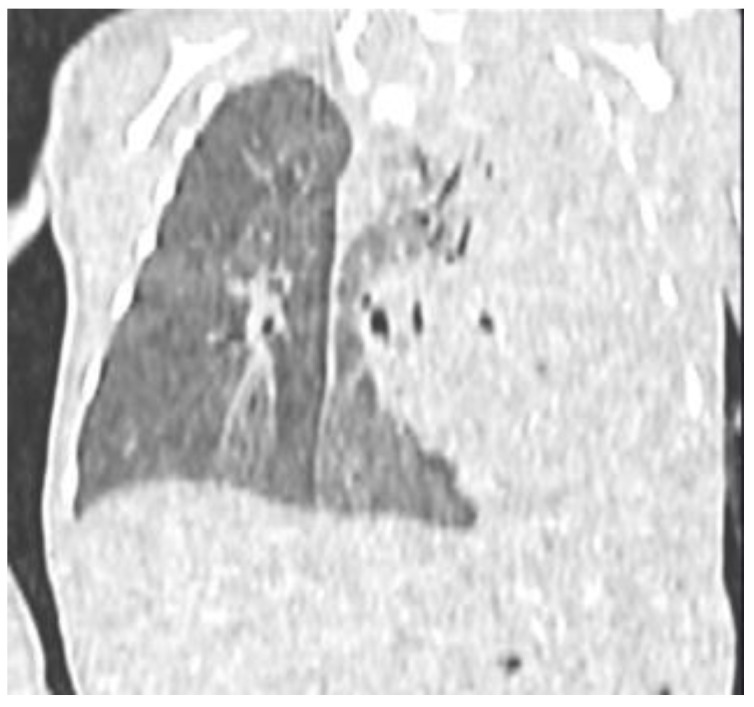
Flash CT scan showing the distribution of the lesion among the majority of the left lung.
